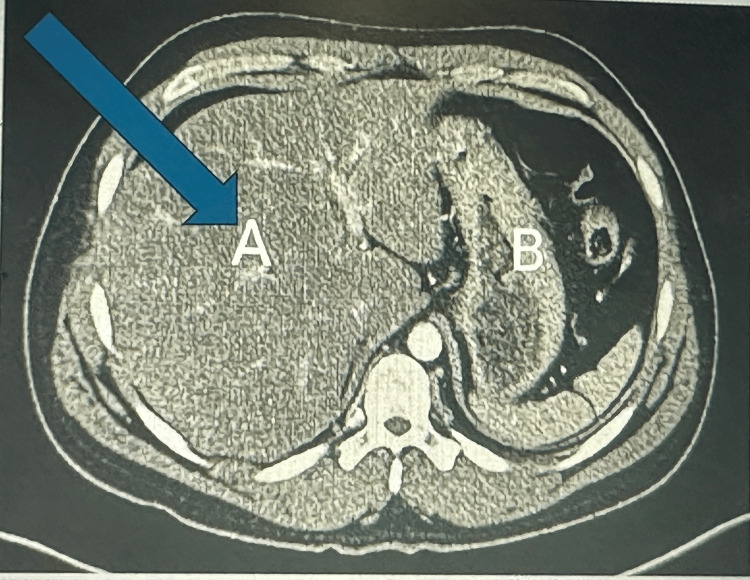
A contrast-enhanced CT scan of the liver showed diffuse low intensity, suggestive of hepatic steatosis. A: The liver shows increased echogenicity and hepatic steatosis; B: Stomach
